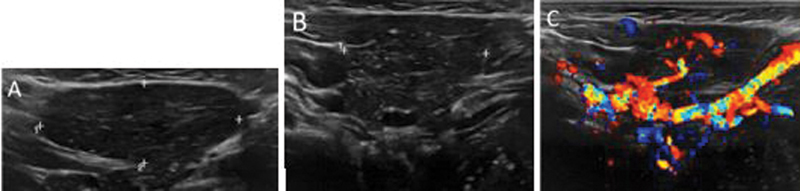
Ultrasound of the submandibular mass demonstrates a mildly spongiform, mildly heterogeneous, hypoechoic internal architecture on sagittal (
